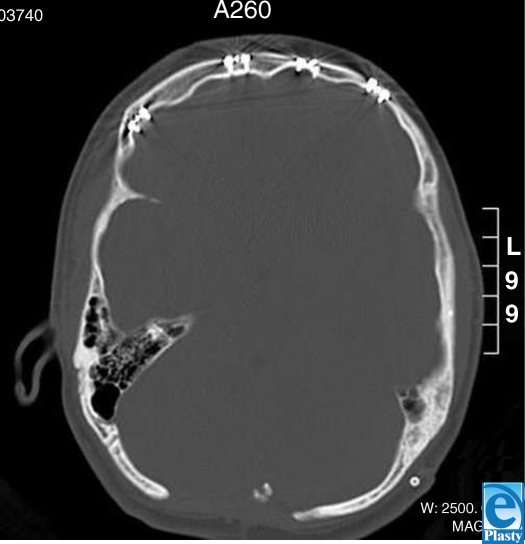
Computed tomography scan and plain film of the patient demonstrating the location of indwelling titanium hardware placed at prior surgery. The burn occurred on the right forehead directly over the most lateral piece of hardware visualized in the coronal section
